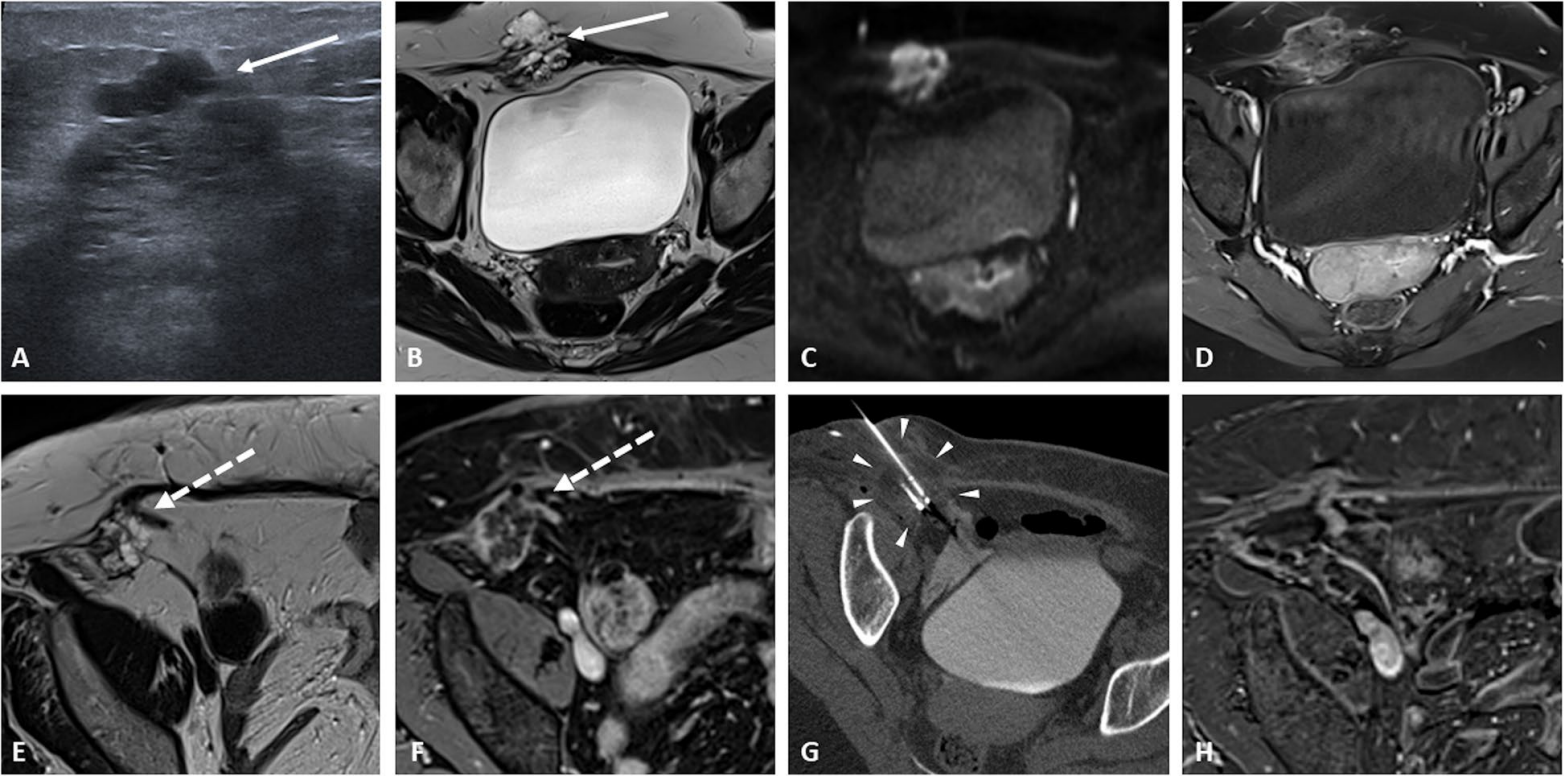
Treatment of an endometriosis-associated clear-cell carcinoma on abdominal wall caesarian scar in a 47-year-old woman. Abdominal wall ultrasound scan (a) shows an ill-defined mass containing cystic portions within the rectus abdominis muscle and subcutaneous fat (solid arrows). Further exploration by MRI with axial T2-weighted image (b) shows a mixed lesion high signal intensity on diffusion image (c high b value) and enhancement of the septae on axial contrast-enhanced fat-suppressed T1-weighted (d). The patient benefited from a complete surgical excision and pathology showed a clear cell carcinoma developed on underlying endometrioma. Two years later, local recurrence is shown on the axial T2 image and fat-suppressed enhanced T1 image (dotted arrows in e and f). Multidisciplinary decision was a conservative percutaneous cryotherapy treatment. The CT scan (g) shows the cryoprobe placement and ice formation during treatment (arrowheads). Follow-up MRI scan at 3 months (h axial fat-suppressed enhanced subtraction image) shows complete disappearance of the mass
